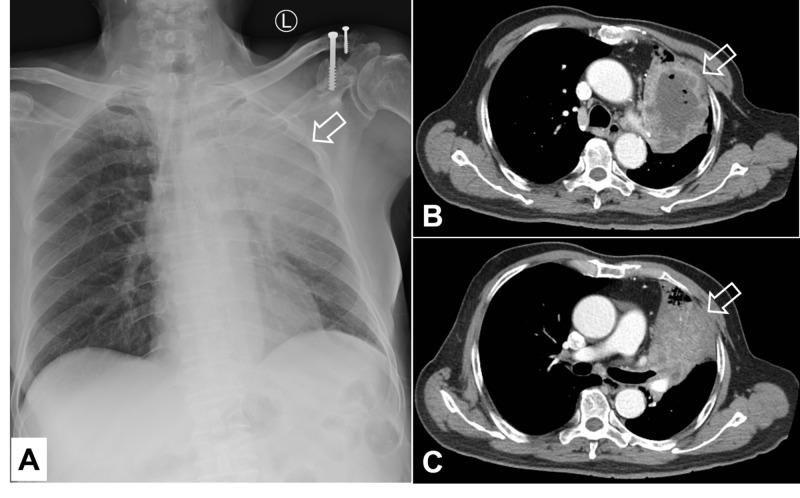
Radiological findings of a massive space-occupying lesion in the upper lobe of the lung at presentation: A. chest X-ray film, B and C. CT scan Arrows: Targets of interest
